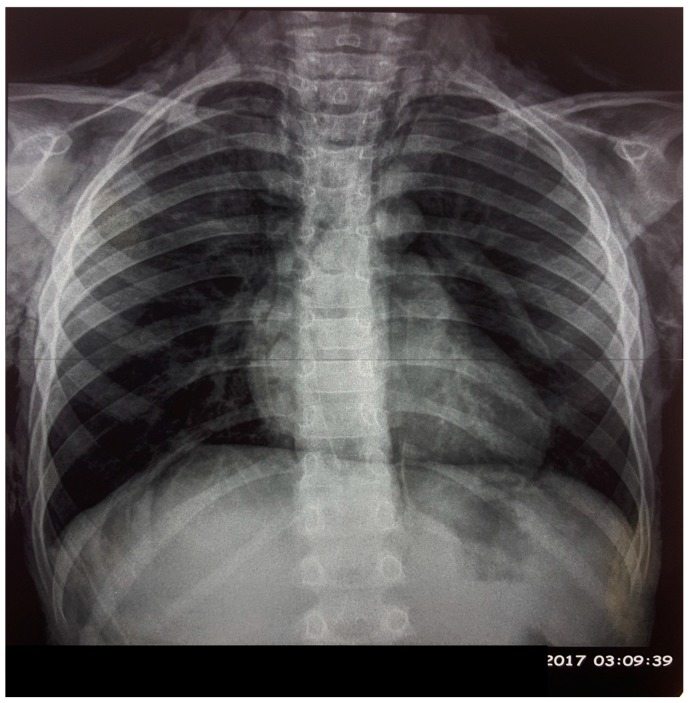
Chest X-ray showing emphysema and pneumomediastinum.
